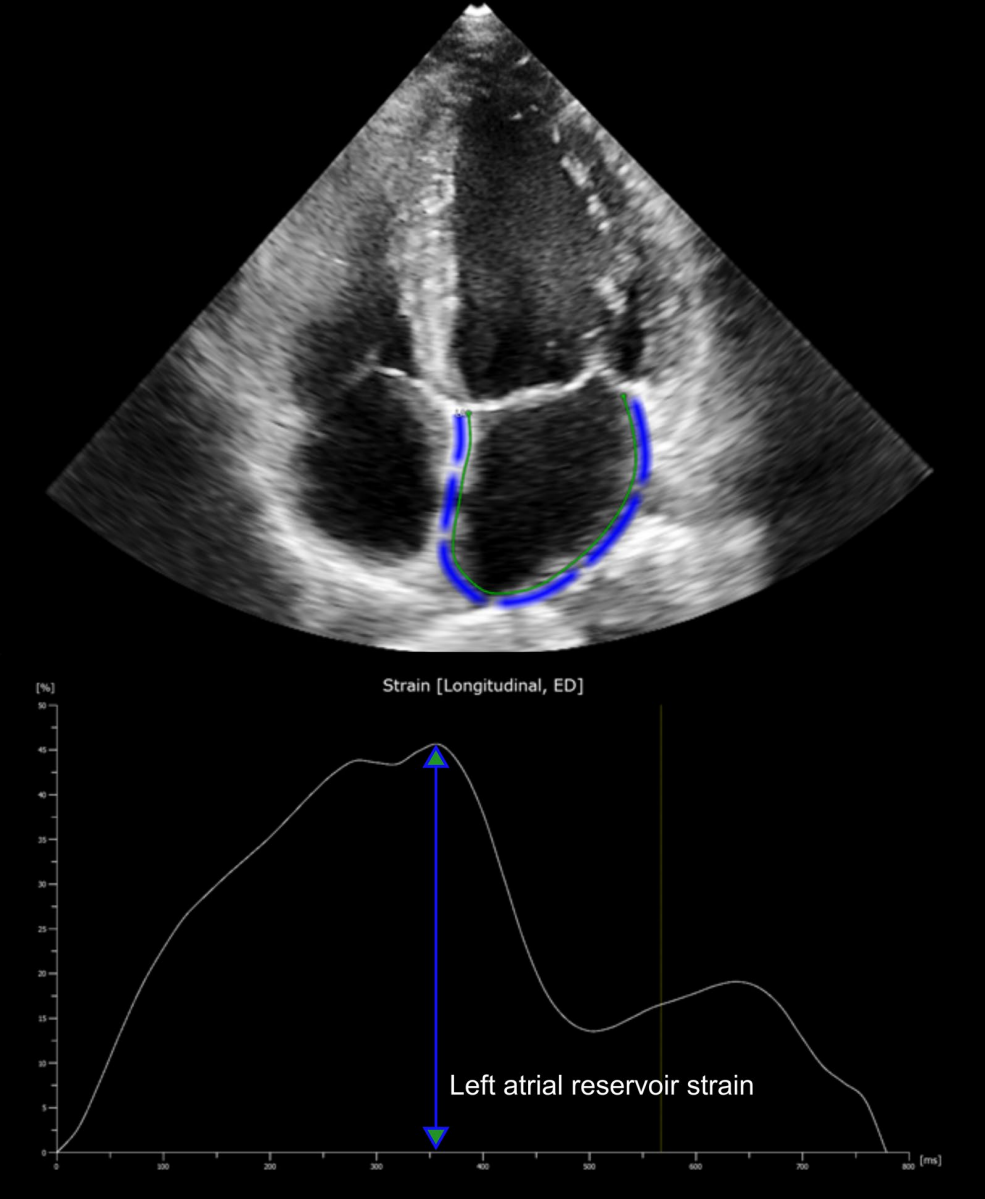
Assessment of left atrial reservoir strain by 2D speckle-tracking echocardiography. This figure shows a non-foreshortened apical four-chamber view of the left atrium. Zero strain reference was set at end-diastole. Arrow represents left atrium reservoir strain (LAS_r_)
